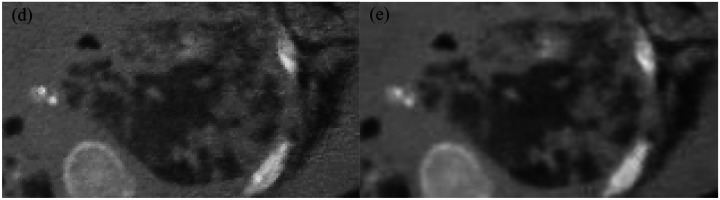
Image reconstruction from the sinogram of 984 views recorded from a clinical CT scanner. (a) The sinogram of 984 views, (b) the image reconstructed using FBP, (c) the image reconstructed using the ISM-based method, and (d-e) enlarged images for red rectangle in images (b-c), respectively.
